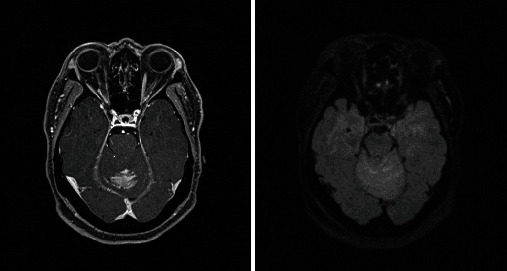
Abnormal contrast enhancing lesion superior cerebellum with surrounding vasogenic edema. Radiographic differential diagnosis: viral or bacterial cerebellitis, autoimmune inflammatory lesion, subacute infarction, and PCNSL.
